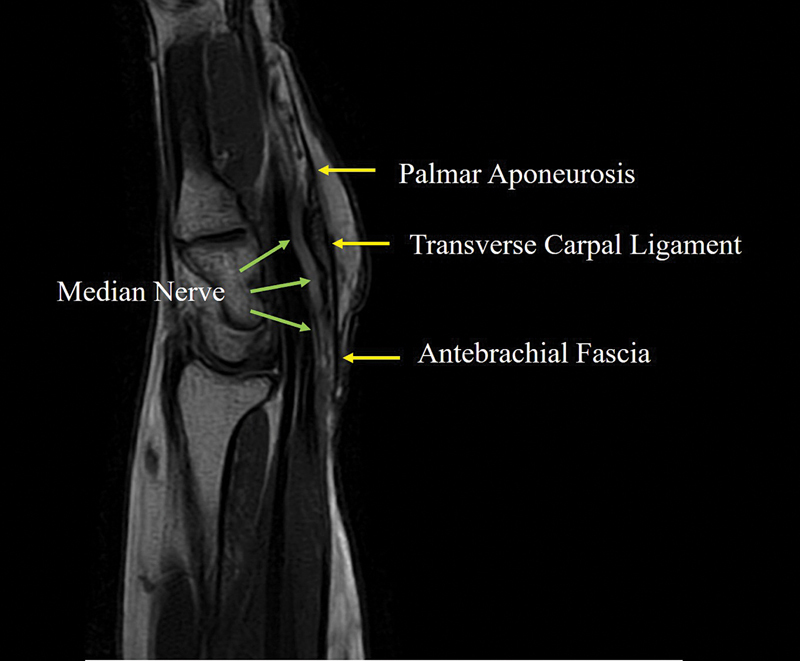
Sagittal MRI scan in severe carpal tunnel syndrome. The palmar boundary of the carpal tunnel is formed by three continuous segments of the flexor retinaculum: (1) a thin proximal segment composed of thickened deep antebrachial fascia of the forearm, (2) the transverse carpal ligament, and (3) a distal portion composed of the palmar aponeurosis between the thenar and hypothenar muscles. MRI, magnetic resonance imaging.
